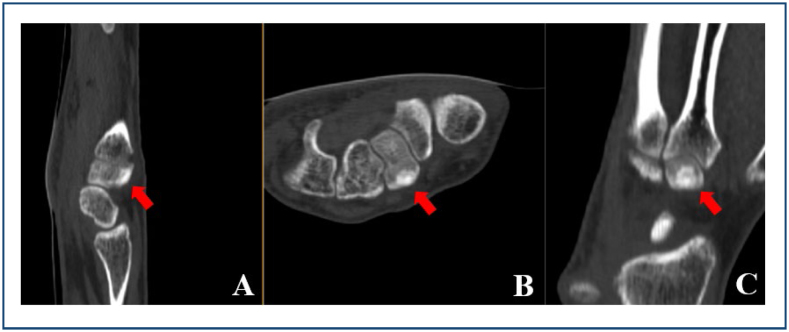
Computed tomography showing a central nidus with surrounding sclerosis in the trapezoid (arrow). (A) Sagittal. (B) Axial. (C) Coronal. Photos cordially provided by Dr. Fábio Augusto Caporrino from Escola Paulista de Medicina, Federal University of São Paulo.
